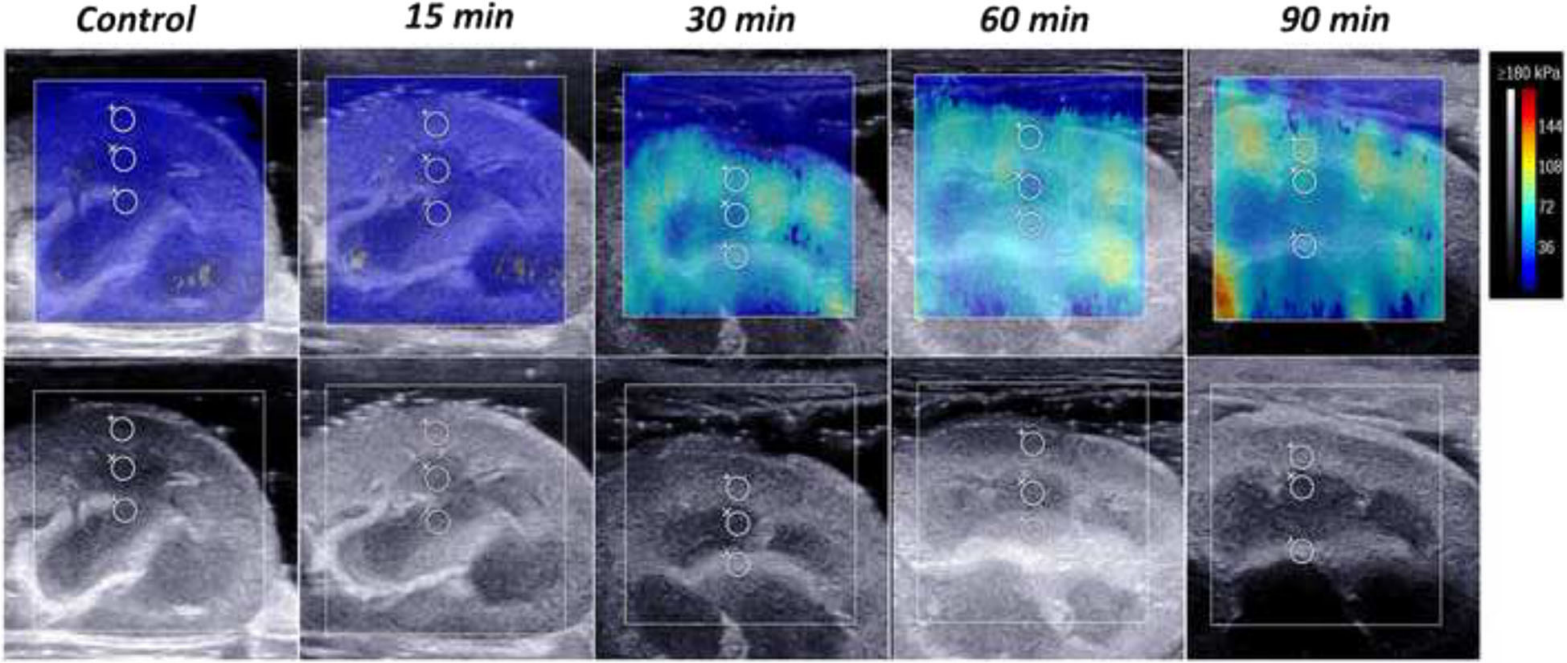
Representative images showing the progressive increase in stiffness by SWE in one kidney when the renal vein was occluded. The lower and upper rows show the changes in the ROI by grey-scale ultrasound and SWE, respectively. The colour map is the distribution of elasticity values scaled from 0 to 180 kPa
